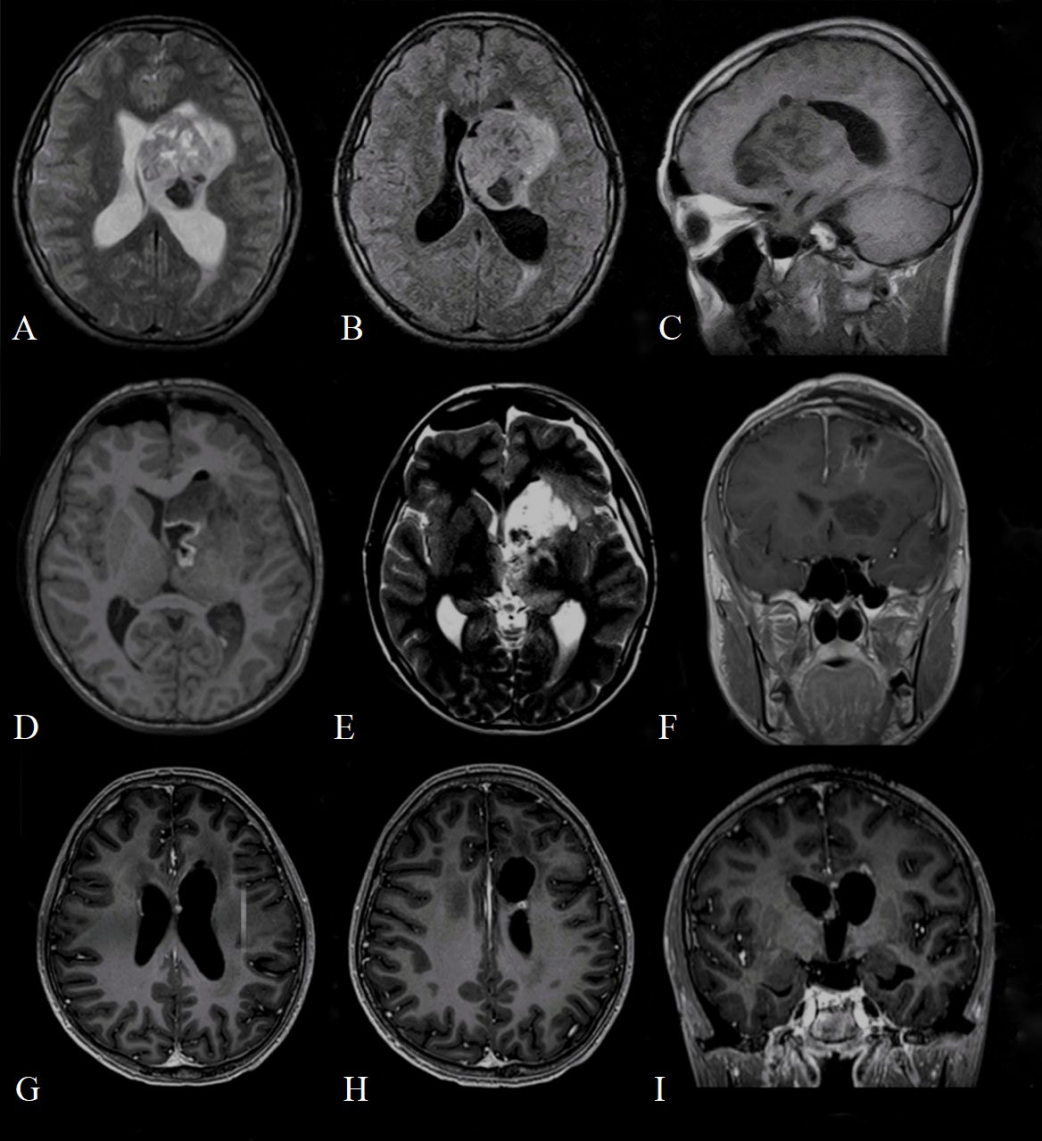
Preoperative (A) T2 axial magnetic resonance imaging (MRI); (B) T2 FLAIR axial MRI; (C) T2 sagittal MRI demonstrating mass in the left lateral ventricle with heterogeneous enhancement measuring 7.2 cm x 4.7 x 5 cm, composed of both cystic and solid areas, and extending to the frontal lobe of the brain; Postoperative (D) T1 axial magnetic resonance imaging (MRI); (E) T2 weighted image axial MRI; (F) T1 contrasted coronal MRI demonstrates the area of postoperative cystic change and residual tumor measuring 1.3 cm x 1.1 x 1 cm, actively accumulating contrast agent; contrasted T1 axial (G, H) and coronal (I) MRI at 17-month follow-up, which shows residual tumor measuring 4.5 x 3.2 mm (H) and 4.3 x 2.6 mm (G).
